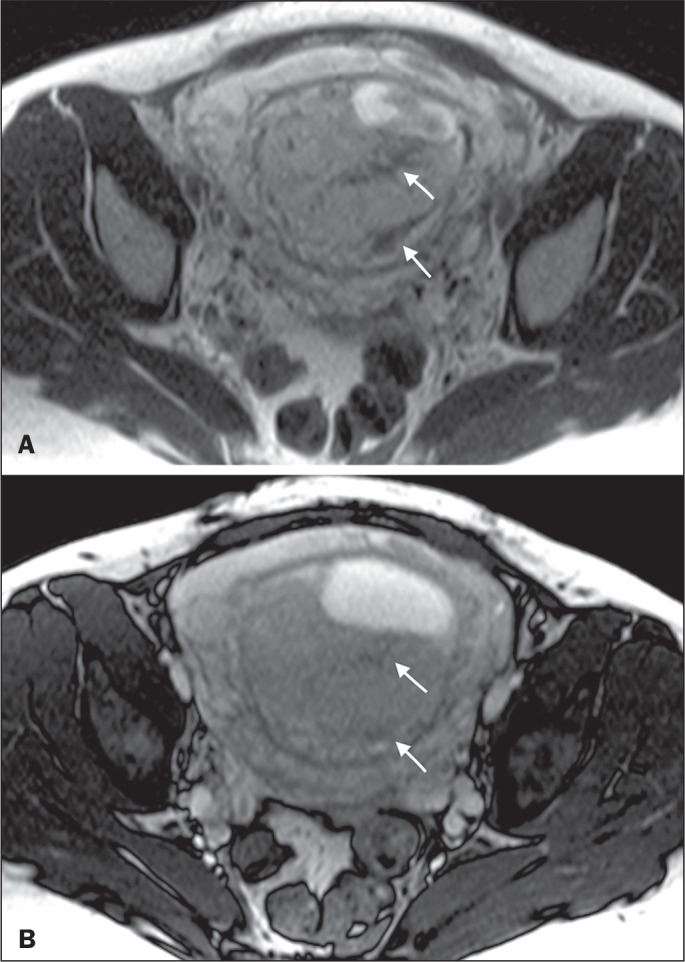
A 30-year-old patient with total placenta previa. Axial T2-weighted HASTE
